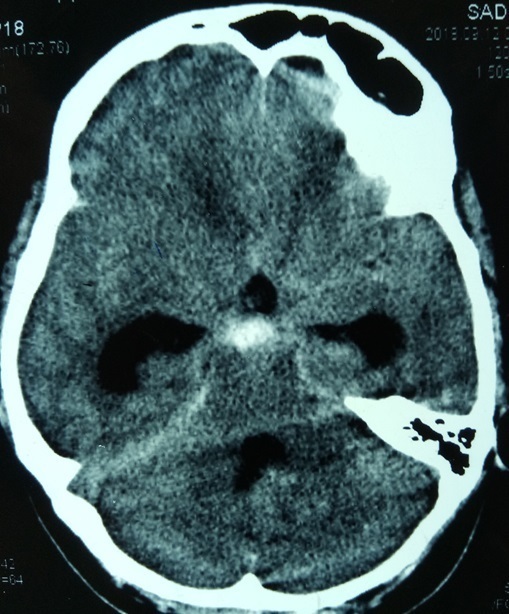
CT scan obtained at admission showing subarachnoid bleed in cisterns with hydrocephalus.
